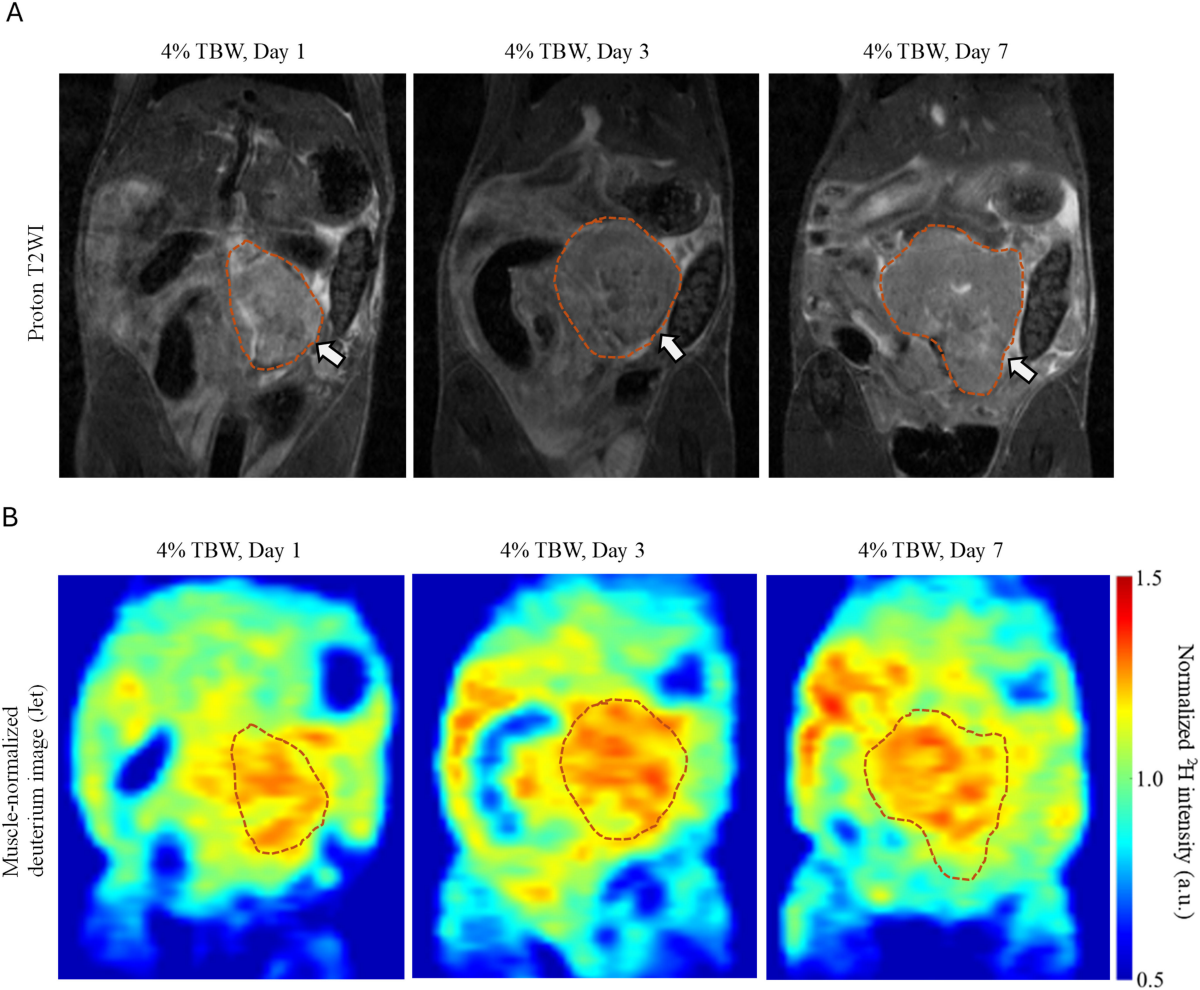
Representative images from the KPC orthotopic model comparing different labeling durations at ~4% ^2^H_2_O in TBW. (A) Coronal proton T2-weighted images: the arrows point to orthotopic tumors delineated with dashed lines in the pancreas. (B) Corresponding muscle-normalized deuterium images did not show significant differences in signal intensity across different loading days, displayed using “Jet” color scale.
